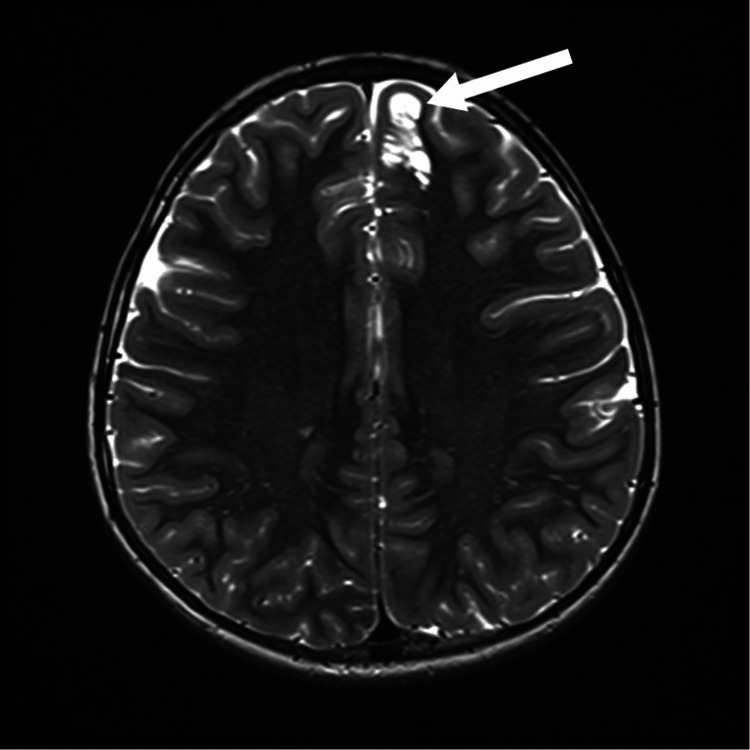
MRI of the brain The described bubbly subcortical lesion in the left frontal gyrus, with multiple septations, sparing the cortex and lacking diffusion restriction and enhancement, most likely represents a multinodular and vacuolating neuronal tumor (MVNT), a benign WHO grade 1 entity, common in pediatric patients. MRI: magnetic resonance imaging
